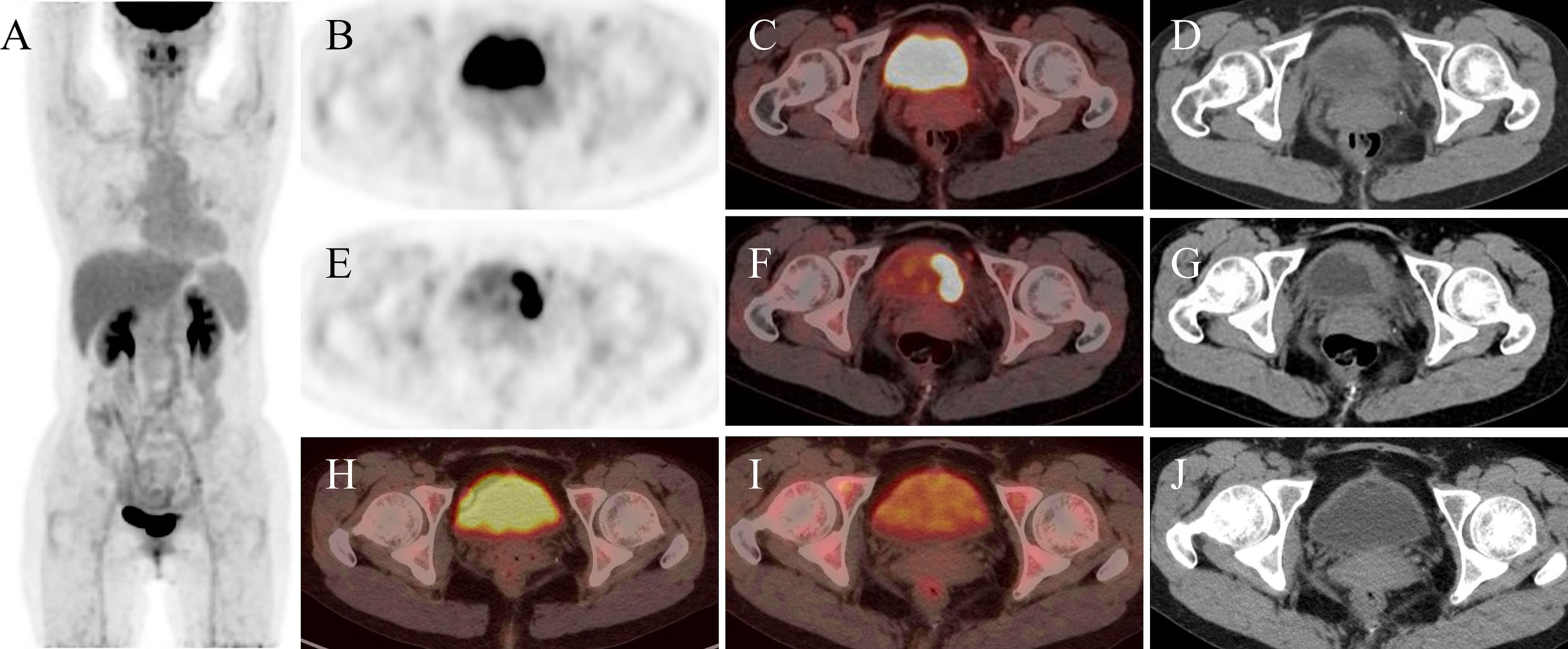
The whole-body ^18^F-FDG PET/CT. Maximum intensity projection (MIP) image prior to chemotherapy (A). Axial PET, PET/CT fusion and CT before furosemide administration (B–D) demonstrate the lesion. Following intravenous furosemide administration, post-diuretic axial PET (E), PET/CT fusion (F), and CT (G) images reveal prominent delineation of the left lateral bladder wall lesion. Post-therapy evaluation after 4 cycles of R-CHOP regimen chemotherapy displays axial PET/CT fusion images of the bladder during standard acquisition (H) and subsequent delayed imaging at 1 h (I), with comparison to pre-chemotherapy post-furosemide images demonstrating notably retained radiotracer activity within the bladder lumen. Corresponding post-chemotherapy CT image of the bladder is presented (J).
